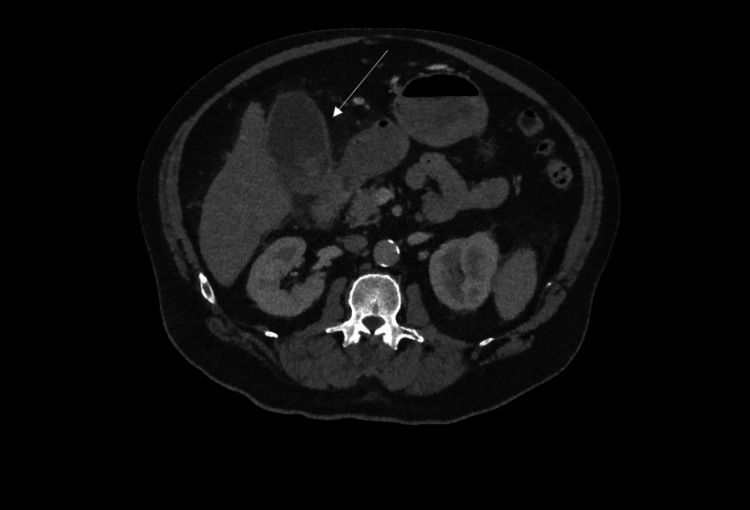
CT abdomen showing a thick-walled, distended gallbladder, in keeping with acute cholecystitis.
